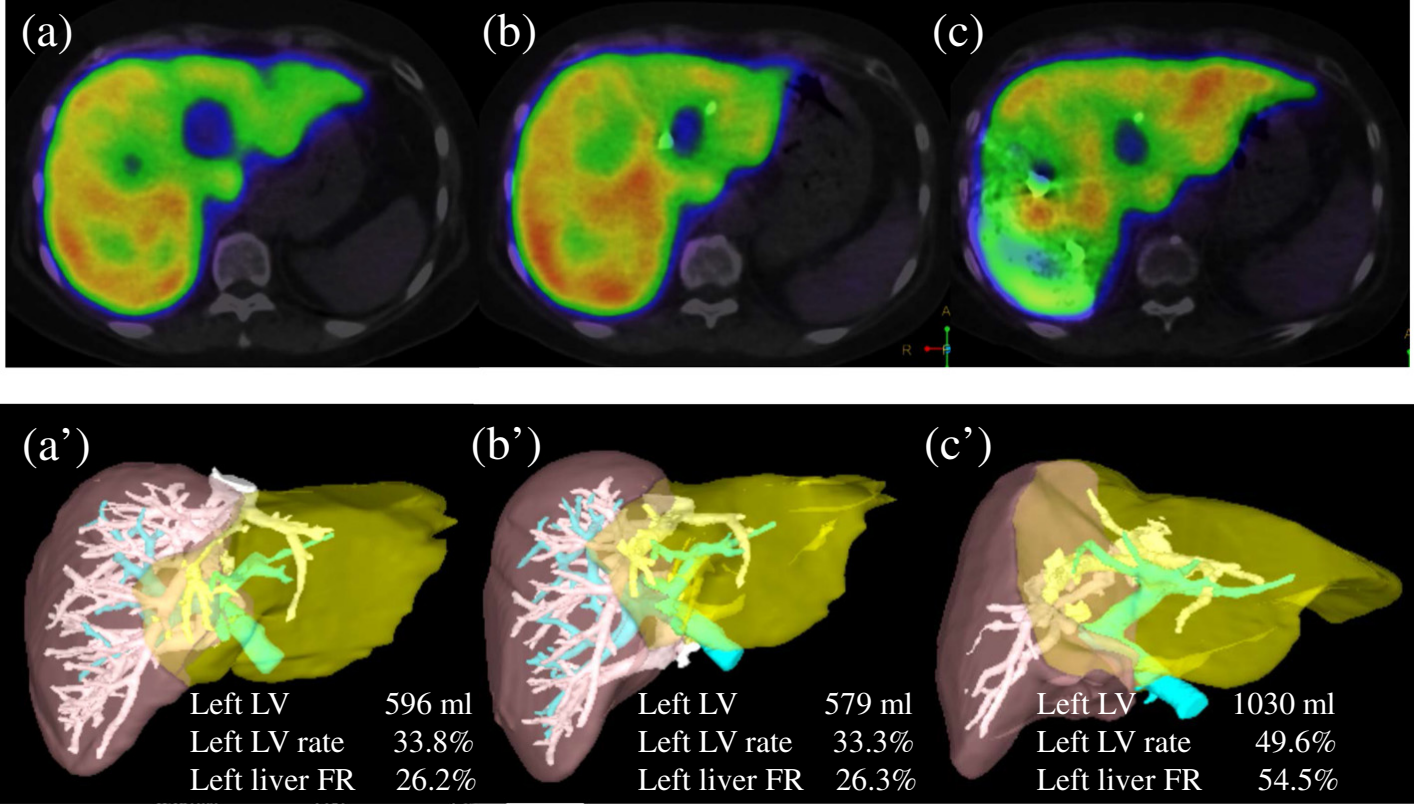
A sequential transition of liver function was shown visually. On admission, ^99m^Tc-galactosyl human serum albumin (^99m^Tc-GSA) single-photon emission computed tomography (SPECT) revealed the superior uptake of hepatocytes in the right liver compared with the left (a), and the volume of the right liver was also greater in quantity on CT volumetry: the left liver volume, the left liver volume rate of the whole liver, and the left liver function rate were 596 ml, 33.8 %, and 26.2 %, respectively (a’). Two weeks after percutaneous transhepatic cholangiodrainage, an extent of the uptake of hepatocytes in the left liver was almost unchanged on ^99m^Tc-GSA SPECT (b), and the volume of the left liver was also stable on CT volumetry: the left liver volume, the left liver volume rate, and the left liver function rate were 579 ml, 33.3 %, and 26.3 %, respectively (b’). After two more weeks following portal vein embolization, ^99m^Tc-GSA SPECT revealed the greatly improved uptake of hepatocytes in the left liver (c), and CT volumetry revealed the swelled left liver almost equal to the right liver: the left liver volume, the left liver volume rate, and the left liver function rate were 1030 ml, 49.6 %, and 54.5 %, espectively (c’). *LV* liver volume, *FR* function rate
